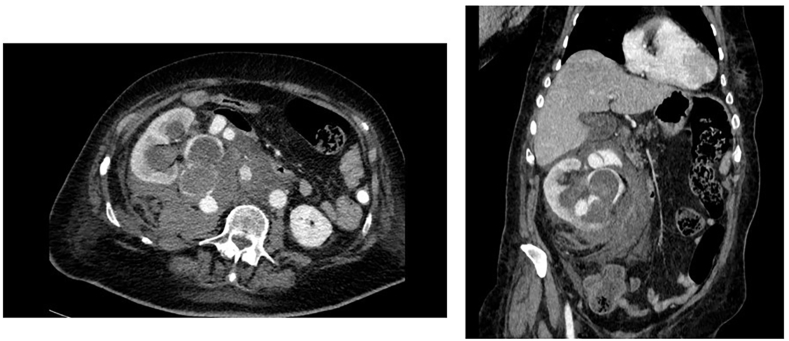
Axial and coronal post rupture CT scan image CT scan image showing retroperitoneal rupture of the right renal arteriovenous malformation and of the excretory tract responsible of a voluminous retroperitoneal hematoma.
